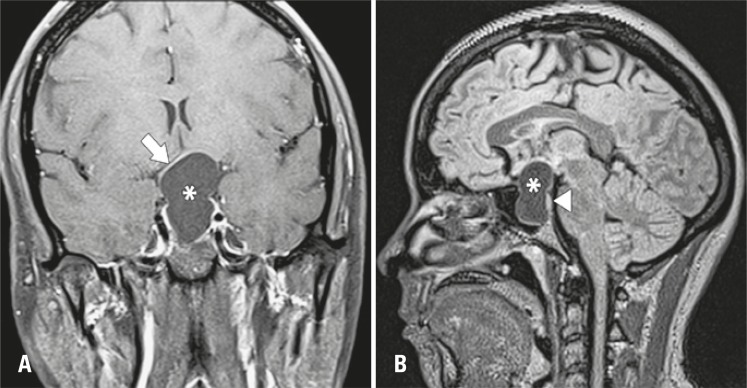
T1-weighted magnetic resonance images. (A) Coronal image after gadolinium administration. The lesion (asterisk) shows thin walls with slight rim enhancement (arrow). (B) Sagittal FLAIR magnetic resonance image. Note the sellar and suprasellar extension of the lesion and the pituitary gland flattened against the *dorsum sellae* (arrowhead)
